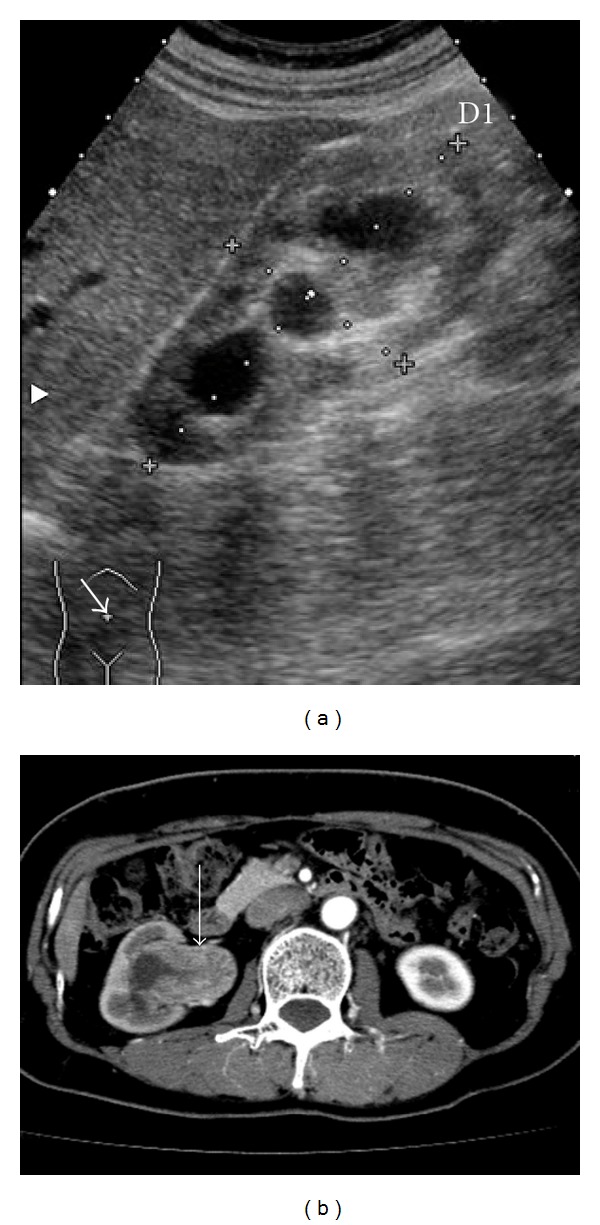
(a) Abdominal echography, showing right-sided unilateral hydronephrosis. (b) Abdominal computed tomography with contrast, showing an enhanced mass in the right renal pelvis (arrow) in association with pelvic dilatation and slight thinning of renal cortex.
